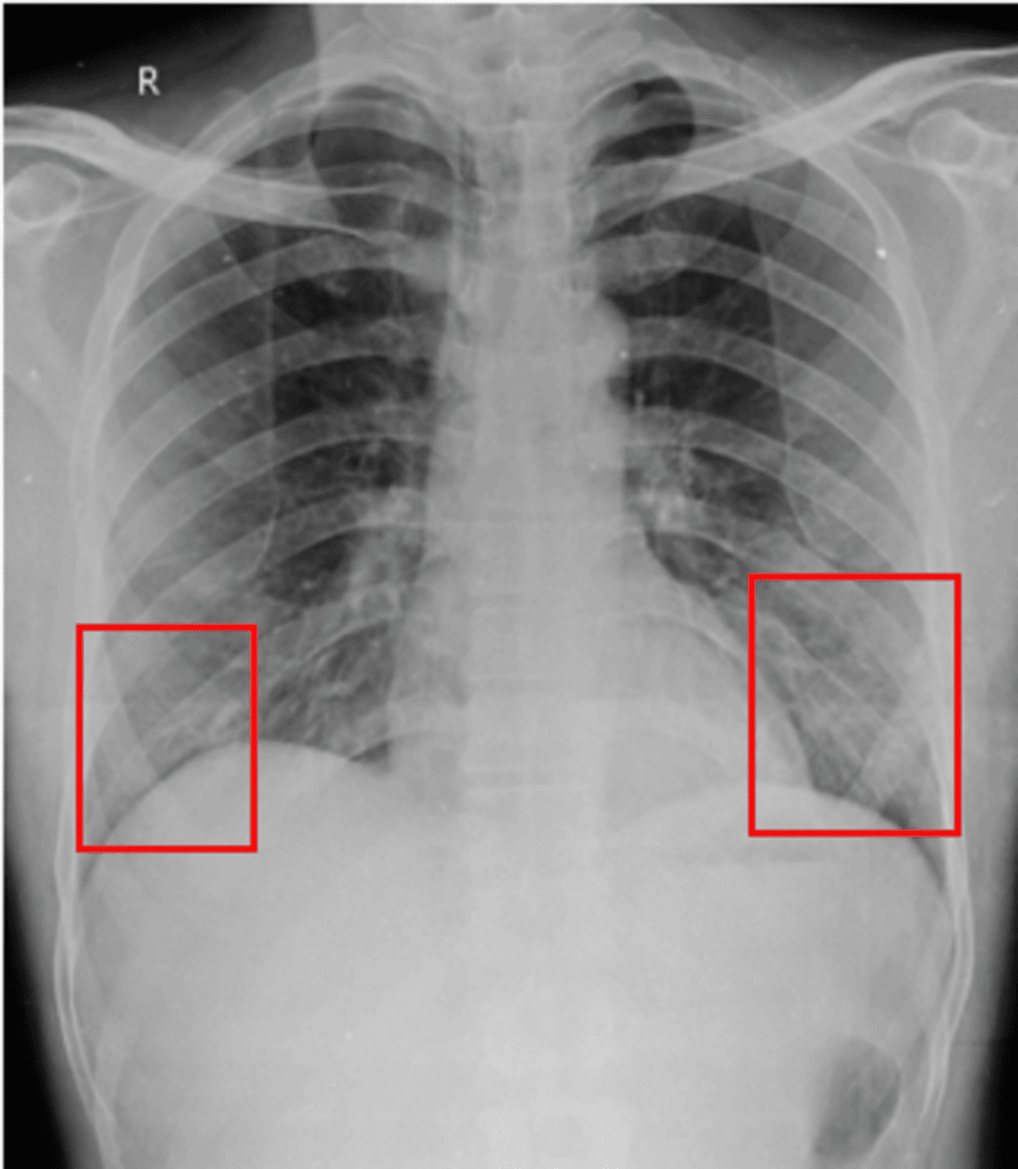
AP view of the chest X-ray taken on day 1 showing bilateral opacity AP: anteroposterior
